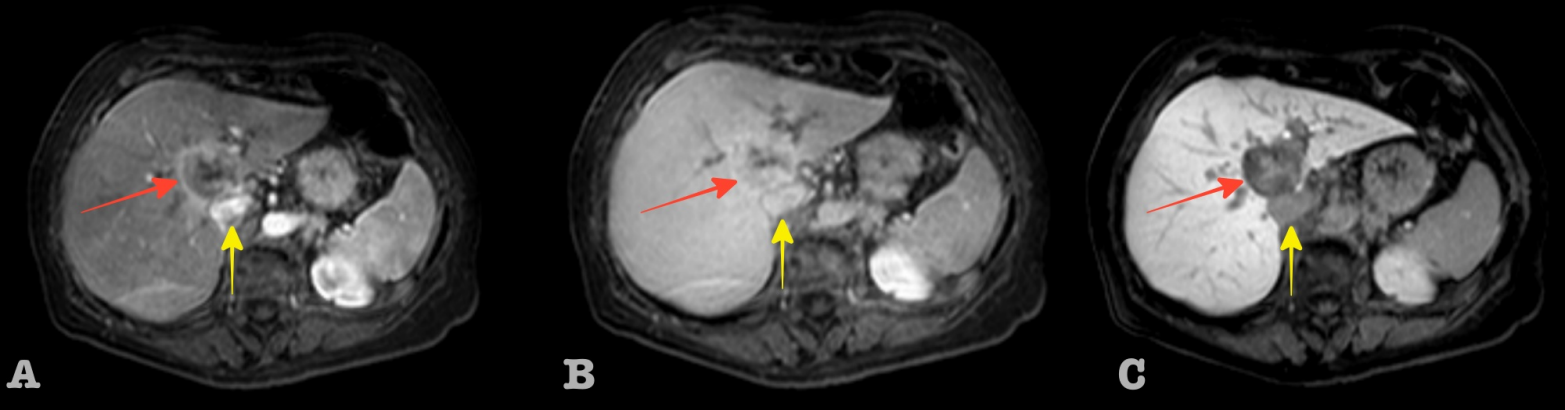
Sequential dynamic contrast enhanced liver MR images. Inflammatory pseudotumor of the liver (red arrow), IVC (yellow arrow): (A) arterial phase 35 sec, (B) portal phase 60 sec., and (C) hepatobiliary phase 30 min. MR: magnetic resonance:  IVC: inferior vena cava
